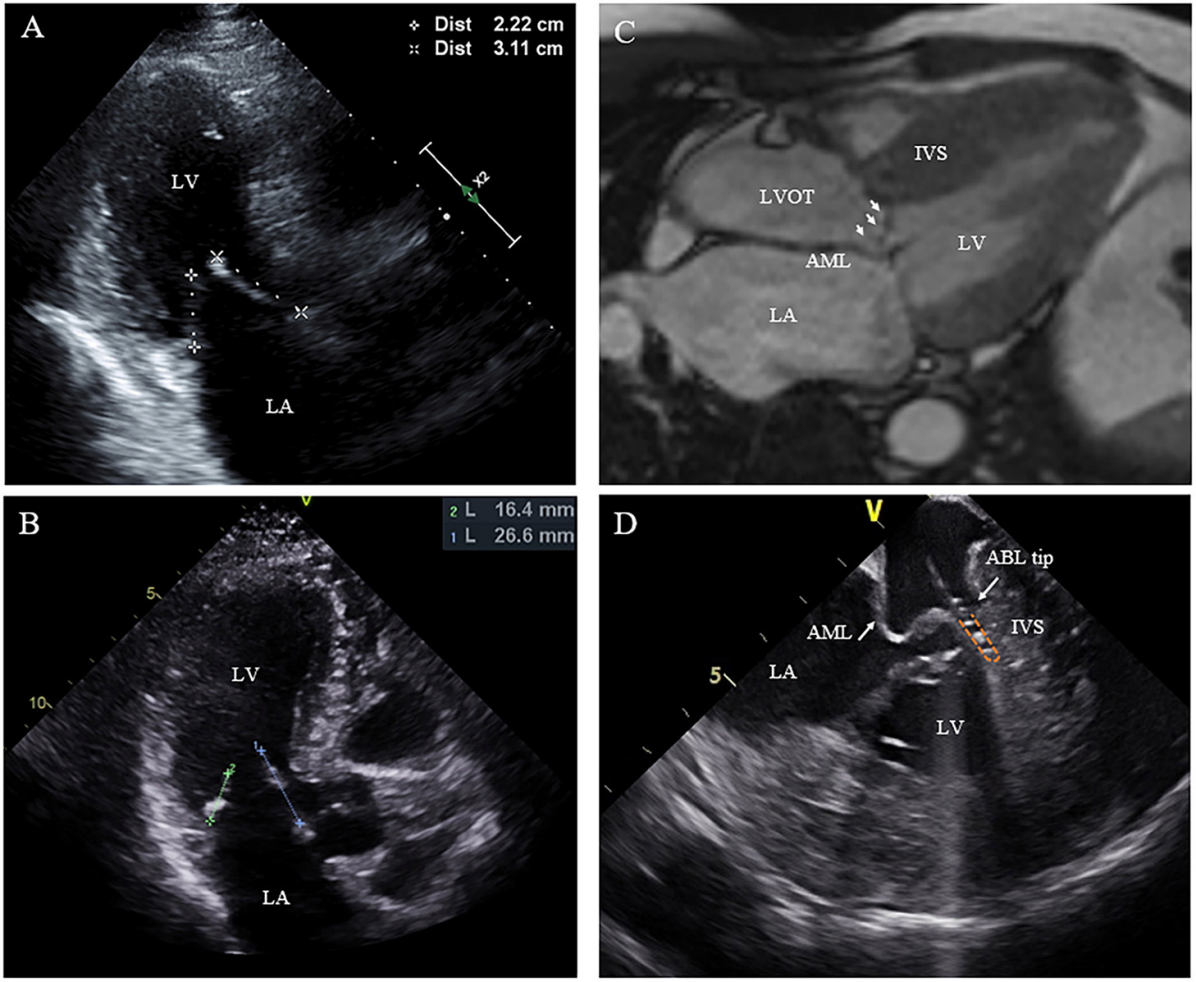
Morphologic abnormalities of mitral valve contributing to outflow tract obstruction. (A,B) The length of the AML was measured by echocardiography in diastole on the apical three-chamber view. (C) Extraordinarily long anterior mitral valve leaflet on CMR. (D) On live ICE screen, the AML (white arrow) is reflexed and attached to IVS during systole and pats on the ablation catheter tip during procedure. AML, anterior mitral leaflet.
